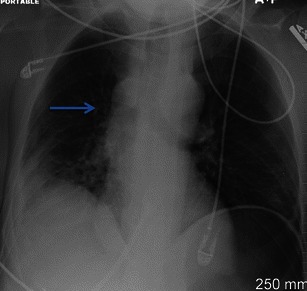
Fluid overload. A 39-year-old female with a history of transfusion after severe abdominal bleeding. AP portable chest X-ray shows an enlargement of the azygos vein (blue arrow) related to fluid overload. In the right lower basal region, a parenchymal consolidation can be seen
